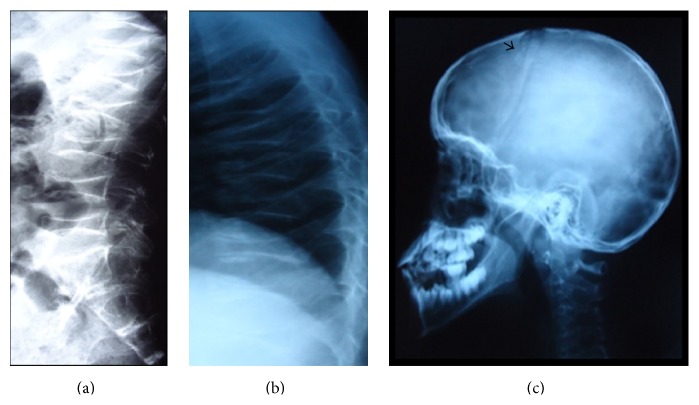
Lateral spine radiographs showing severe osteopenia, platyspondyly (a, b), Skull X-ray revealed wormian bone ↘ (c).
